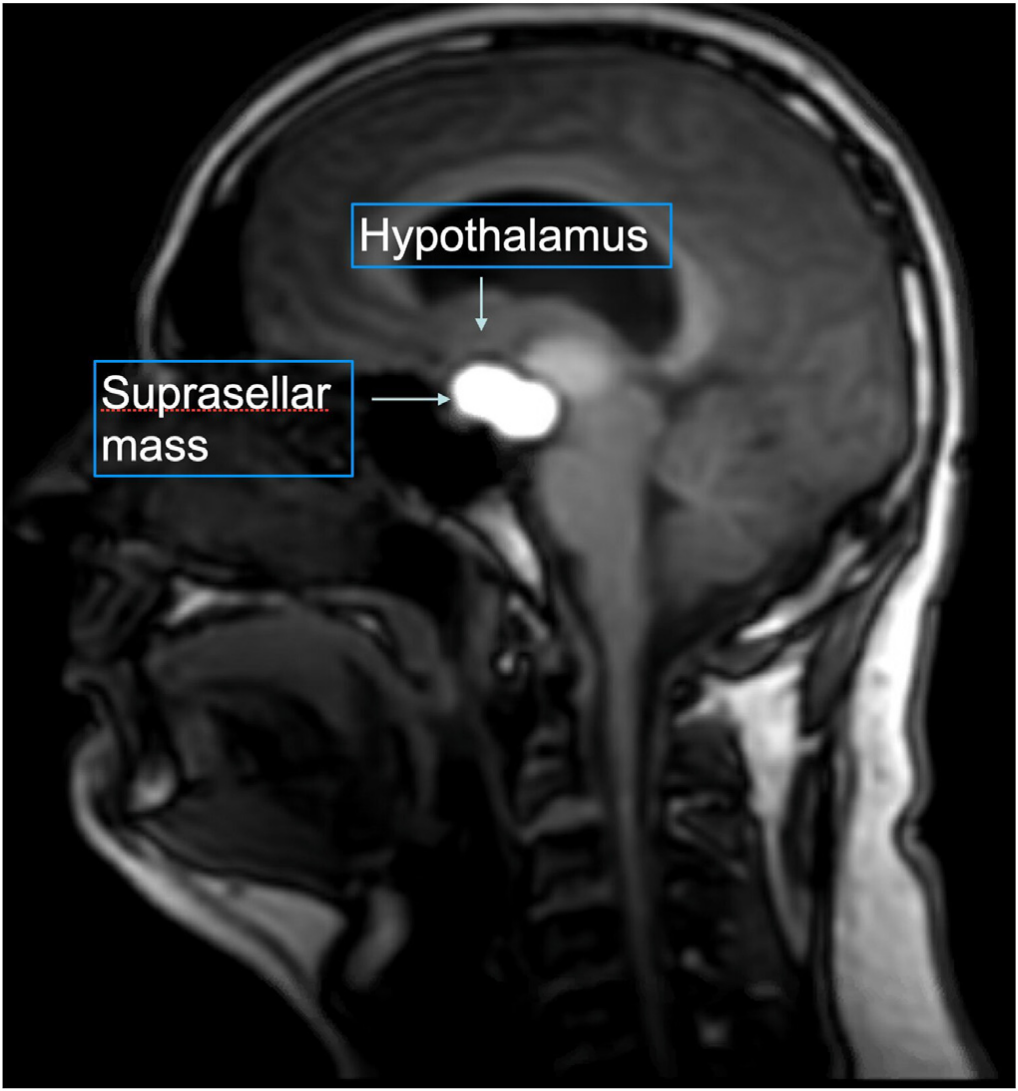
Magnetic resonance Images of the brain without and with intravenous gadolinium using multiplanar multisequence imaging. Large, predominantly cystic sellar and suprasellar mass measuring approximately 4.7 × 2.7 × 3.6 cm (anteroposterior × transverse × craniocaudal), with enhancing solid components and multiple fluid-filled levels, including layering hemorrhage. Mass effect was exerted upon surrounding structures, including the optic chiasm, third ventricle, bilateral posterior and anterior cerebral arteries, as well as the right cavernous and supraclinoid internal carotid artery. Hydrocephalus with mild periventricular T2/fluid-attenuated inversion recovery hyperintensity.
